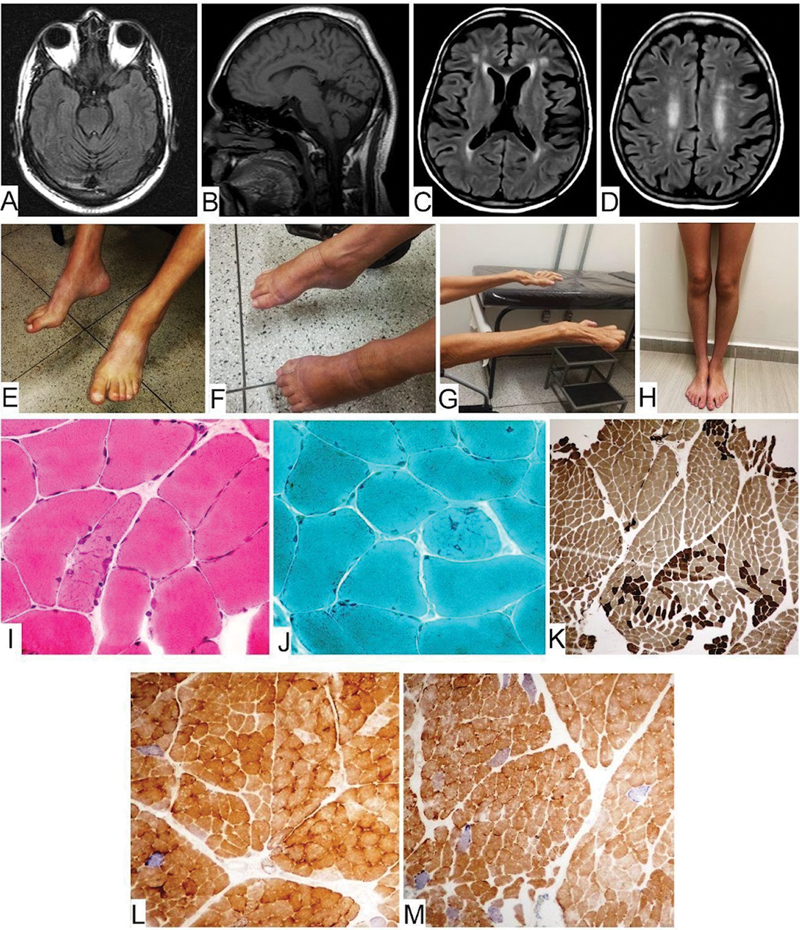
Brain magnetic resonance imaging (MRI) scan showing cerebellar atrophy, affecting predominantly the lobes: fluid-attenuated inversion recovery (FLAIR) sequence (
